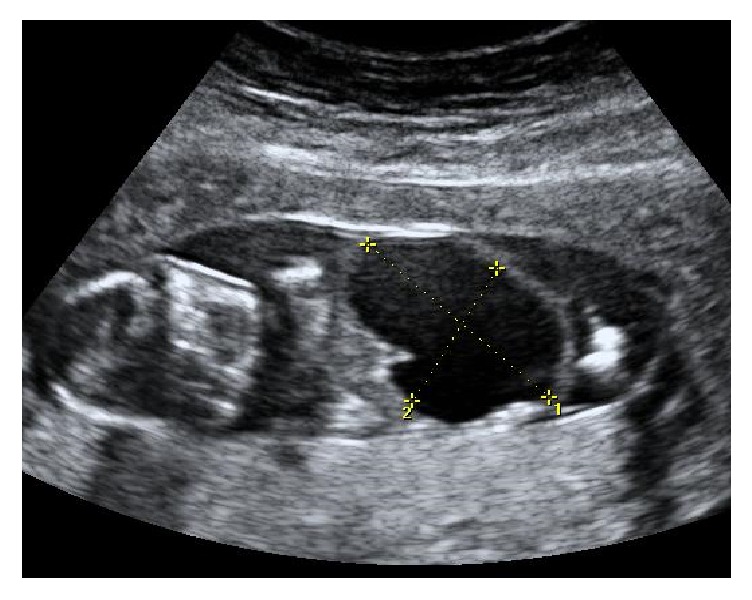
Ultrasonographic findings of the fetus at 14 weeks of gestation. A fetal intra-abdominal cyst (34 × 24 mm in size) and oligohydramnios are observed.
